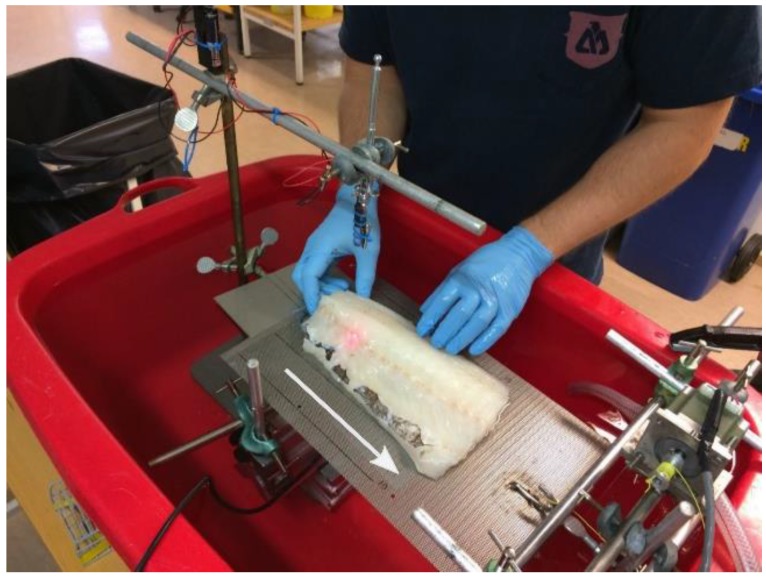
Complete setup placed in a plastic tub for immersion in water. The fish fillet in placed on a perforated plate with a slit in the middle to allow the ultrasound to pass through to the fillet. The plate is pulled by a stepper motor in the direction of the white arrow. A laser pointer mounted above the fillet indicates the position of the transducer focal point, enabling the operator to aim the ultrasound at the bone row during continuous treatment.
